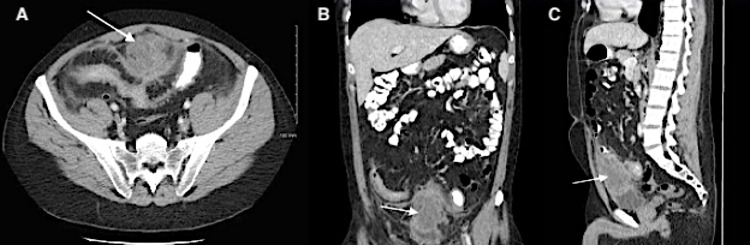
Computed tomography of abdomen/pelvis demonstrating an intraabdominal abscess (arrows) secondary to complication of Crohn’s disease in axial (A), coronal (B), and sagittal (C) planes.
